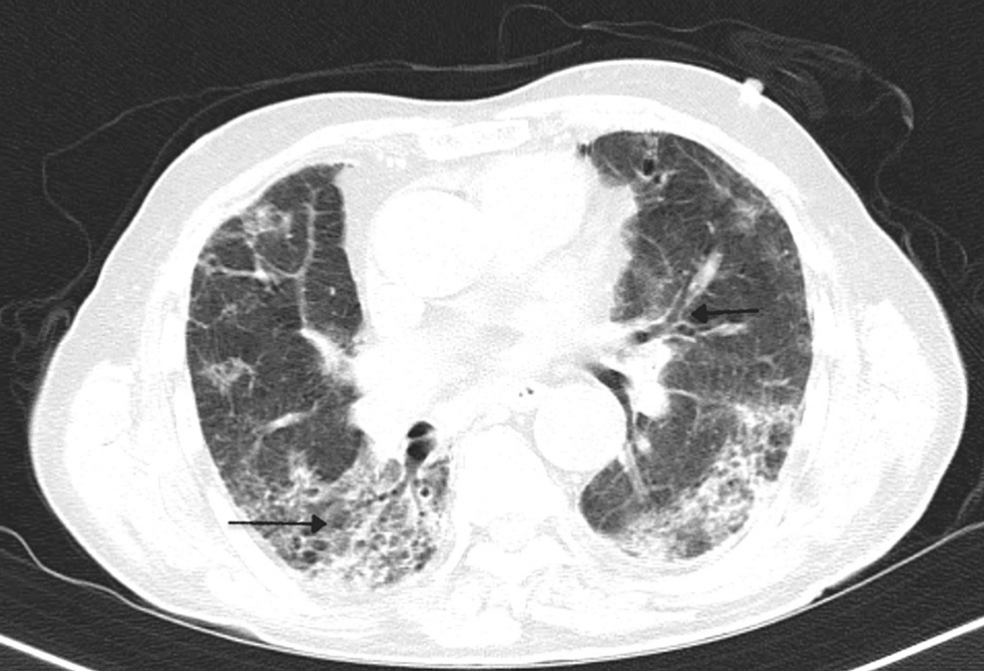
HRCT thorax image showing ground-glass opacities with patchy areas of consolidation (left arrow) and traction bronchiectasis (right arrow) HRCT: high-resolution computed tomography
